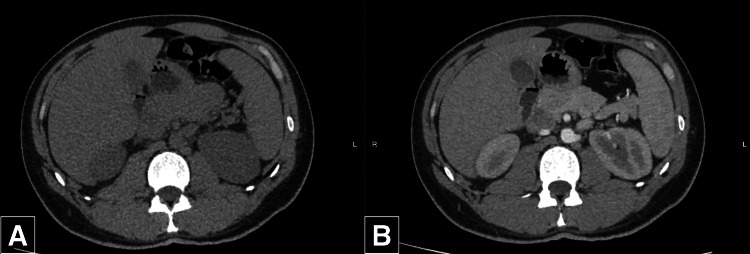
Abdominal CT scan for evaluation of potential liver abscess Panels A and B show non-contrast and contrast-enhanced abdominal CT scans, respectively. No signs of lesion of the hepatic parenchyma can be seen, and all other abdominal structures are normal.
